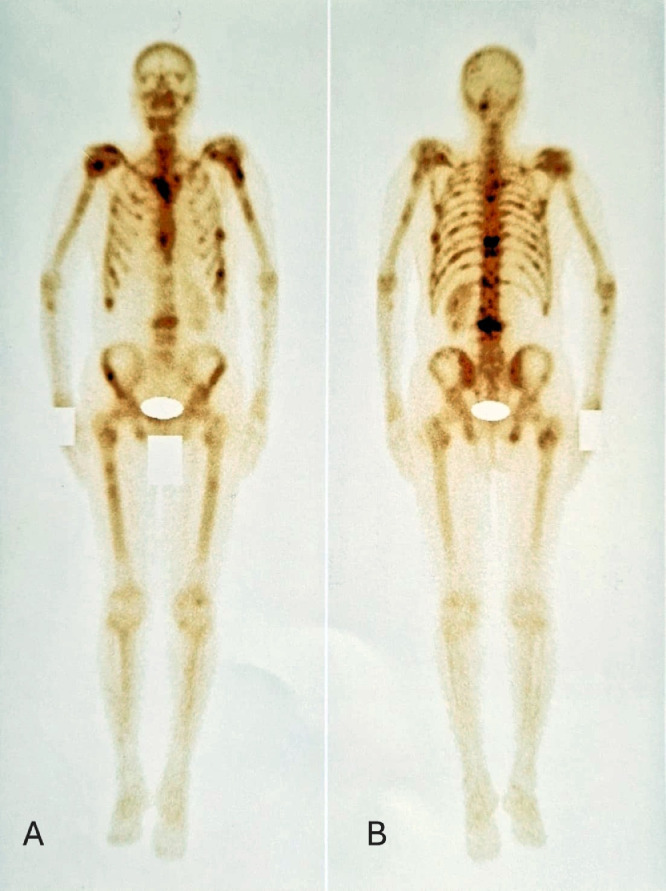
Whole-body bone scintigraphy showing anterior (A) and posterior (B) projections with multiple foci of increased tracer uptake consistent with bone metastases.
